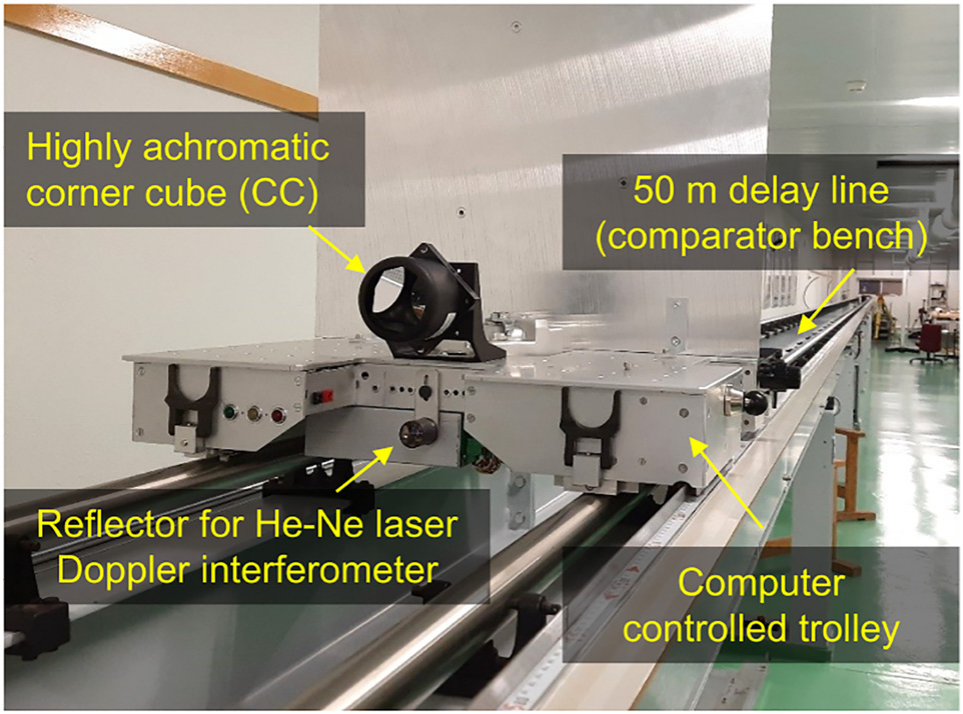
Picture of the computer-controlled trolley on a linear 50 m long comparator bench, along with the reflectors for SC-based EDM and He–Ne laser Doppler interferometer.
